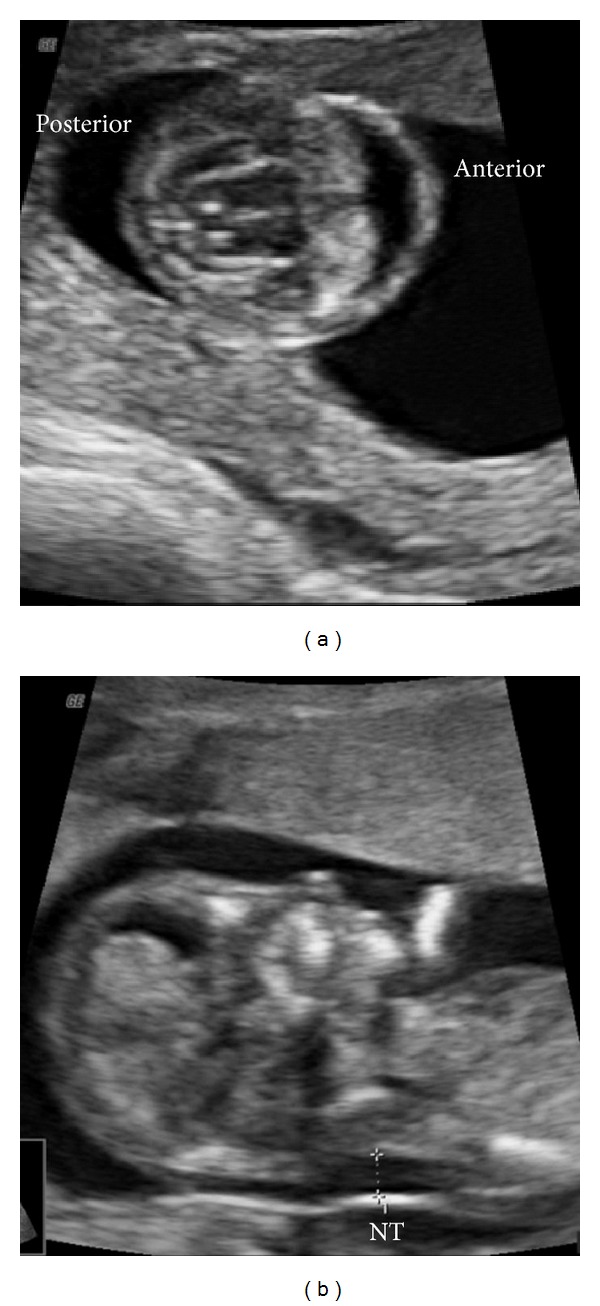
Ultrasound images at 12 3/7 weeks gestation. (a) Transverse view of the fetal intracranial anatomy demonstrates a single anterior ventricle and fused thalami; (b) sagittal view of the fetal profile demonstrates an increased nuchal translucency (NT) of 2.9 mm and a flattened profile with absent nasal bone.
